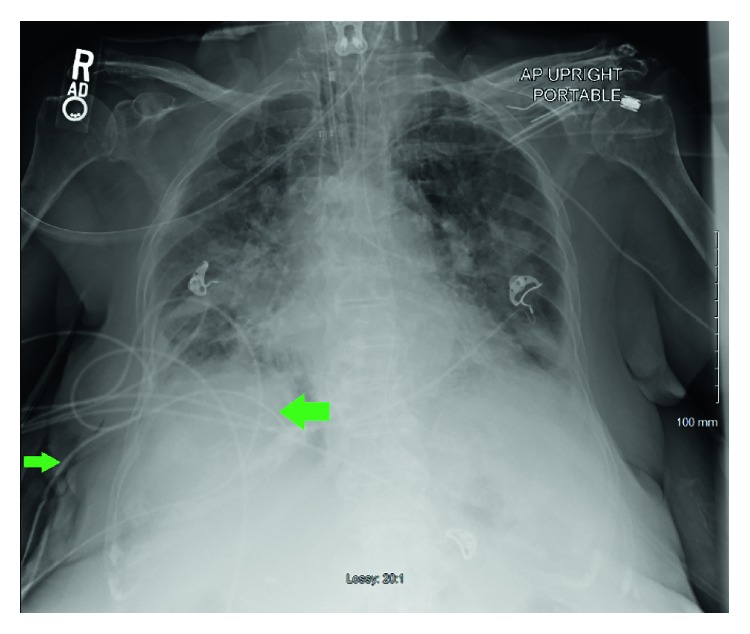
Chest X-ray showing the right-sided chest tube (green arrows), positioned in the most dependent area.
